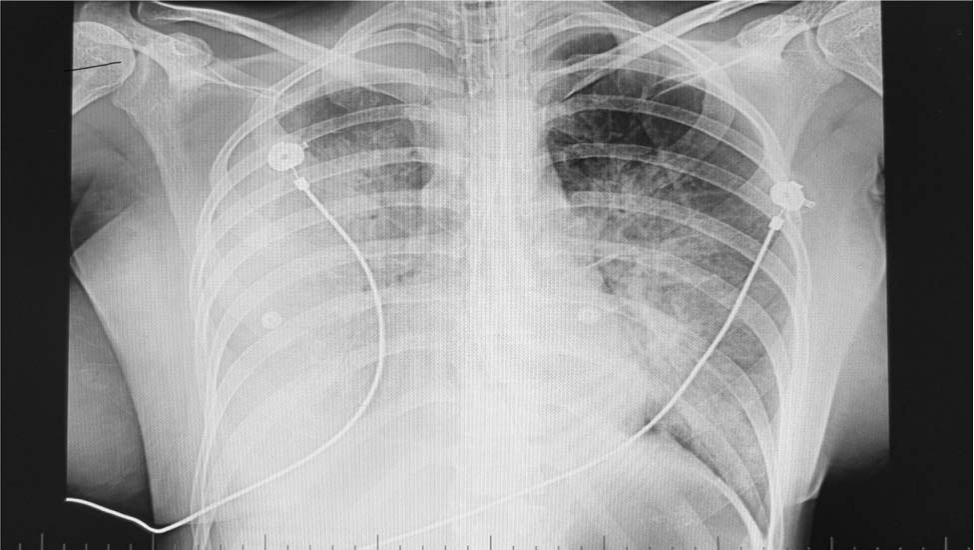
Chest x-ray finding on the third day of admission.
